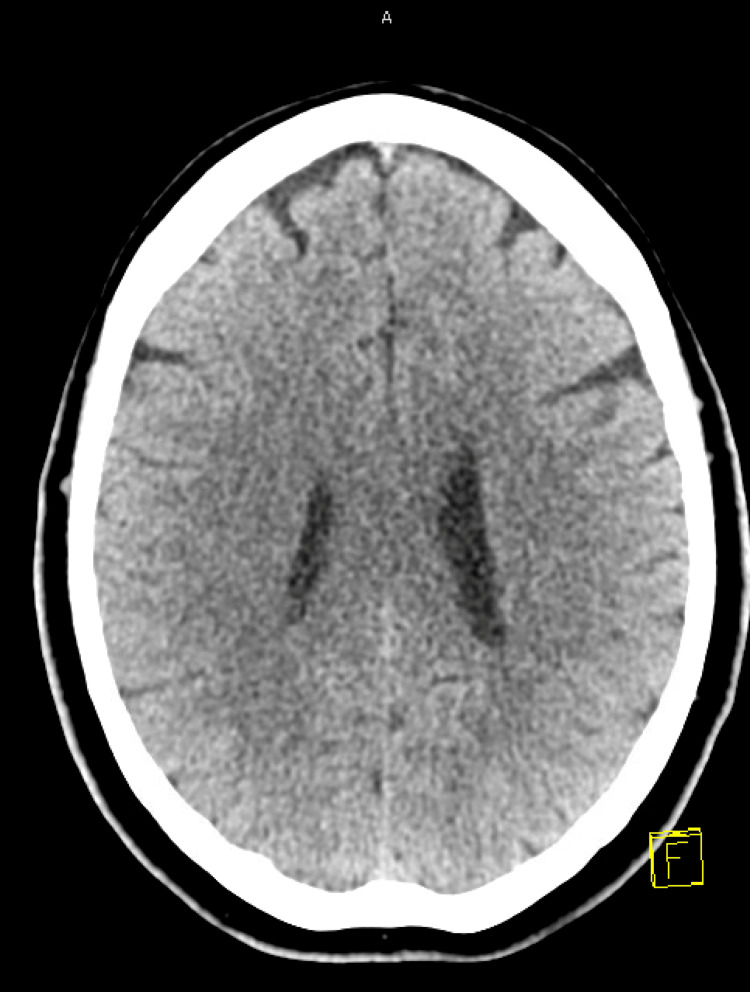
Normal CT Head CT: Computed Tomography
